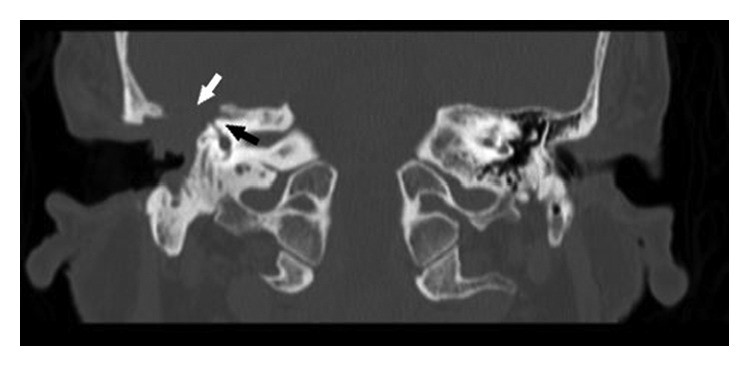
Coronal section of temporal bone CT. A mass obliterates the outer ear pathway and destroys the mastoid cells and mastoid antrum. The mass extends to the middle ear. The mass was caused by ossicular chain and tegmen tympani defects. White arrow: Tegmen tympani defect. Black arrow: defect in superficial semicircular canal.
